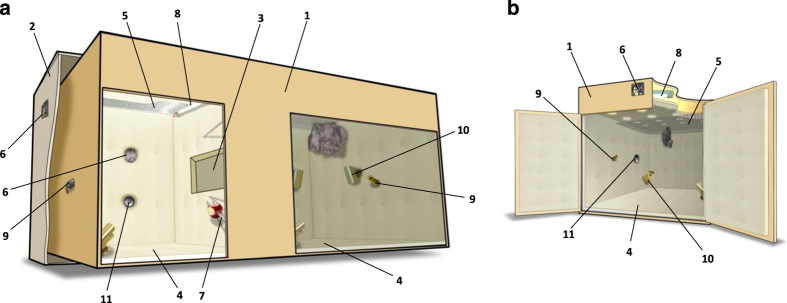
The recording setup. (a) *Colony* chamber (length: 190 cm; width: 90 cm; height: 82 cm). Chambers of this type housed *Colony* treatment adults and pups, as well as groups of weaned pups in both treatments. (b) *Isolation* chamber (length: 120 cm; width: 70 cm; height: 60 cm). Chambers of this type housed *Isolation* treatment mothers with their pre-weaned pups. Legend: 1. wooden box; 2. outer box for acoustic isolation; 3. A window allowing transition between the ‘roost’ compartment and the ‘foraging’ compartment, and allowing some light from the ‘foraging’ compartment to penetrate the ‘roost’ during the day; 4. foam for echoes reduction; 5. plastic mesh for facilitating hanging from the ceiling; 6. airflow ventilators; 7. feeding skewers; 8. lights (active during daytime); 9. ultrasonic microphones; 10. infra-red sensitive video cameras; 11. loudspeakers (not used in this study).
